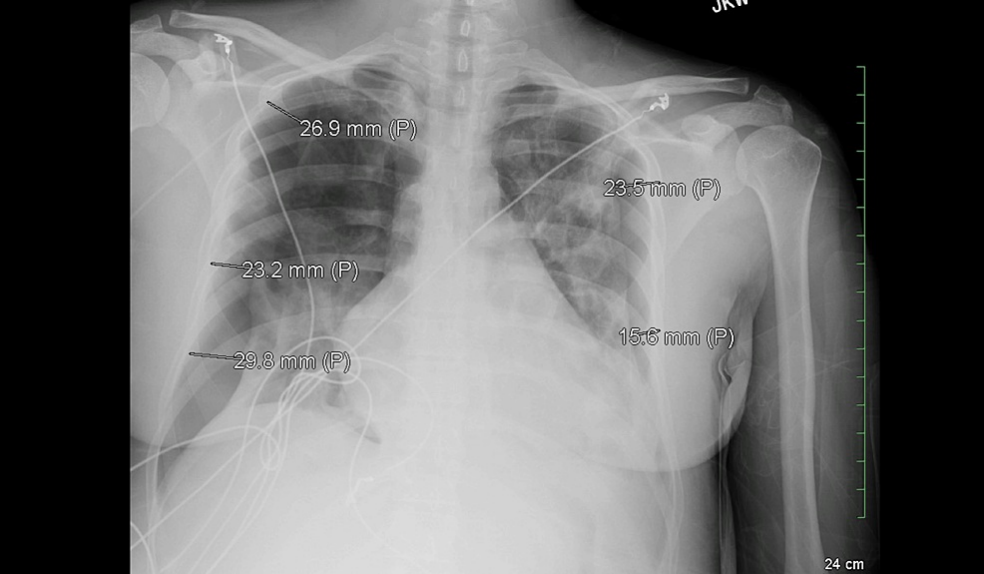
Chest X-ray showing newly developed bilateral pneumothorax. The numbered markings in the above figure show the intrapleural distance between the parietal and visceral pleura that is used by radiologists to calculate the Rhea index to classify the size of pneumothorax as small, moderate, or large [4]. This was labeled as a moderate-sized pneumothorax.
